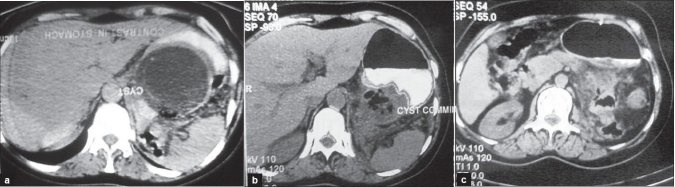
(a) Computed tomography of the abdomen showing the retrogastric cyst compressing the contrast-filled stomach. (b) CT of the abdomen showing the retrogastric cyst emptying into the contrast-filled stomach. (c)CT of the abdomen showing complete evacuation of the cyst
